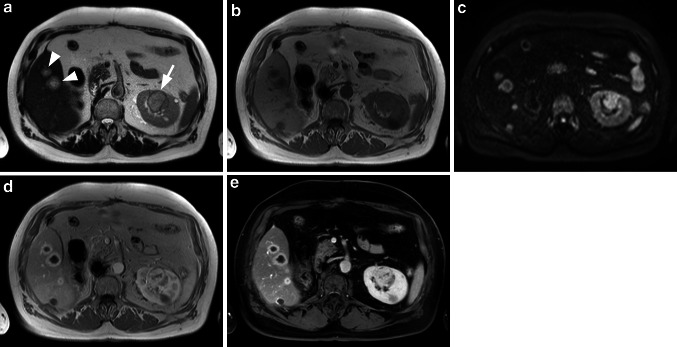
RCC with multiple liver metastases. The T2w HASTE sequence (a) reveals multiple liver metastases (*arrowheads*) from a RCC of the left kidney (*arrow*). On the corresponding T1w image (b) the liver lesions are hypointense to the liver parenchyma, whereas the RCC is isointense with the renal cortex. On the DWI image with a *b*-value of 800 (c) the liver metastases as well as the tumor in the left kidney are hyperintense indicating diffusion restriction. After injection of 0.05 mmol/kg gadobenate dimeglumine, the liver lesions as well as the RCC of the left kidney are hypervascular during the early arterial phase (d) with the RCC showing early contrast wash-out on the T1w fs image (e) during a later phase
